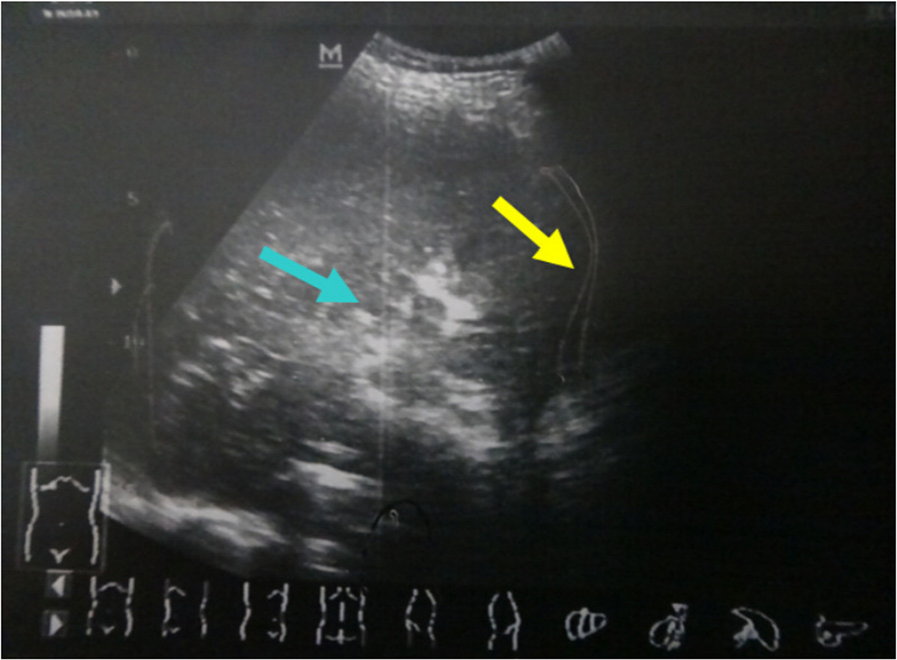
Color doppler ultrasound investigation. The ultrasound showing fluid around liver and hilus lienis (yellow arrow) and uneven right liver lobe (blue arrow). The gallbladder cannot be detected
